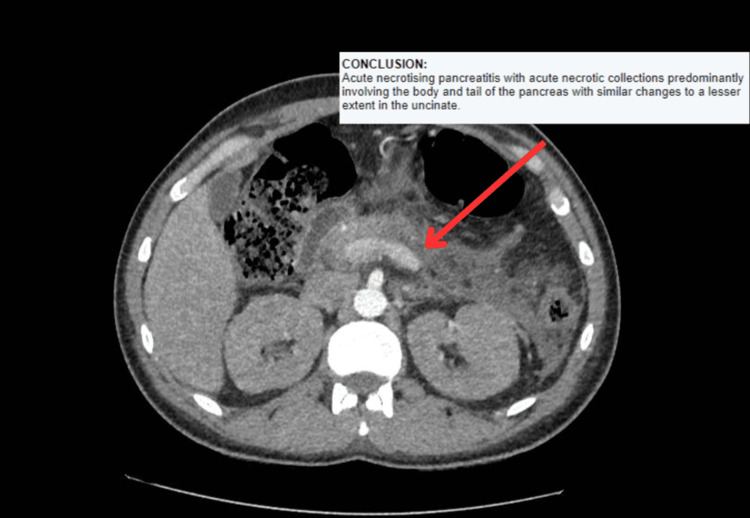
CT of the abdomen showing acute necrotizing pancreatitis.
